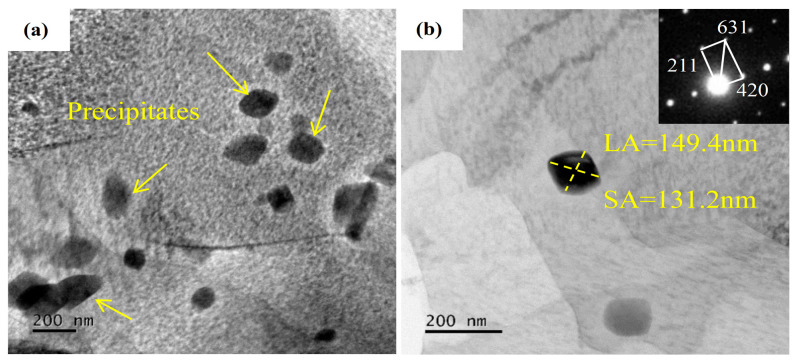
The TEM images of precipitates of A210-CT sample. (a) precipitates, (b) precipitate size and identification.
